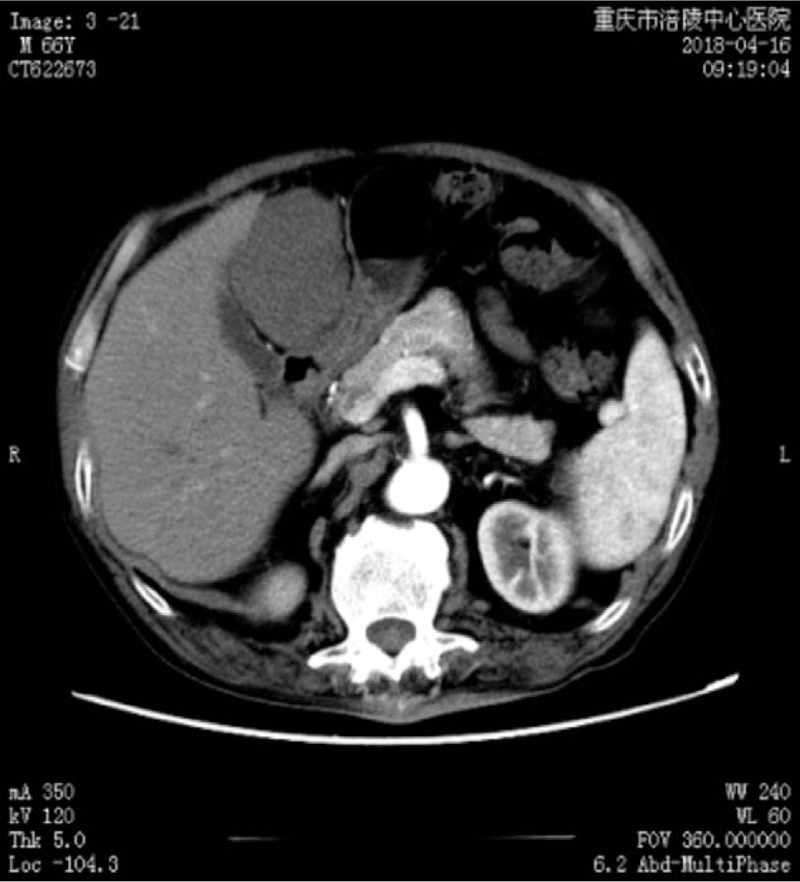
Computed tomography scan of the abdomen. The mixed echoic mass measures 10 × 8 × 8 cm and is located below the liver, adjacent to the gastric antrum. There is no calcification in the mass, and no contrast after injection of a contrast medium.
